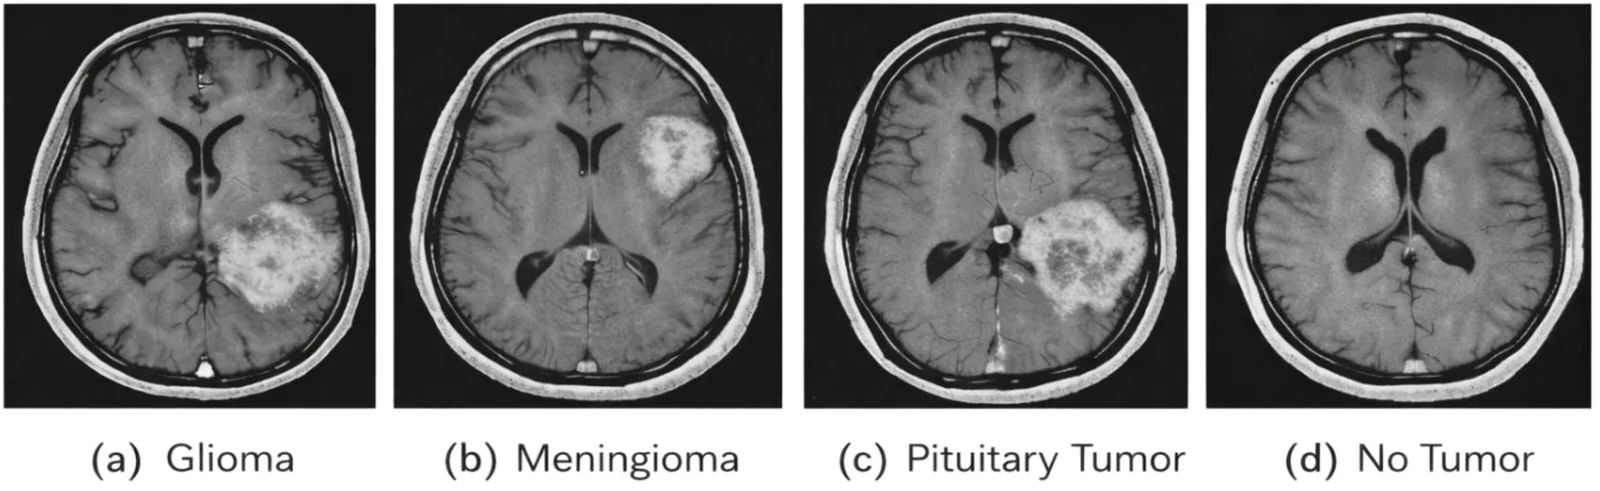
Sample MRI images of (a) glioma (b) meningioma (c) pituitary tumor and (d) no-tumor.
